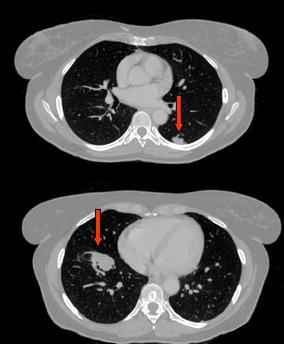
Bilateral lung metastases at CT-scan
